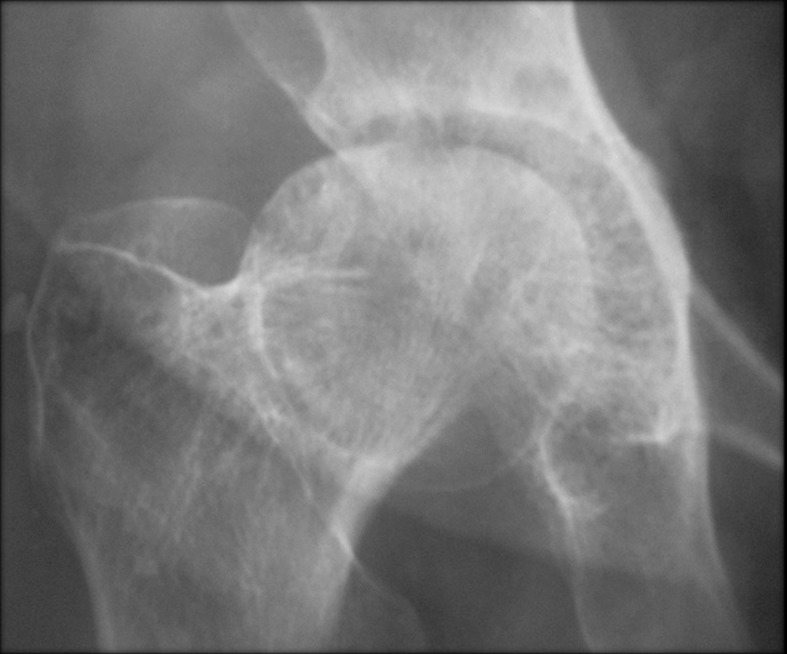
aseptic necrosis of the hip on radiographs
